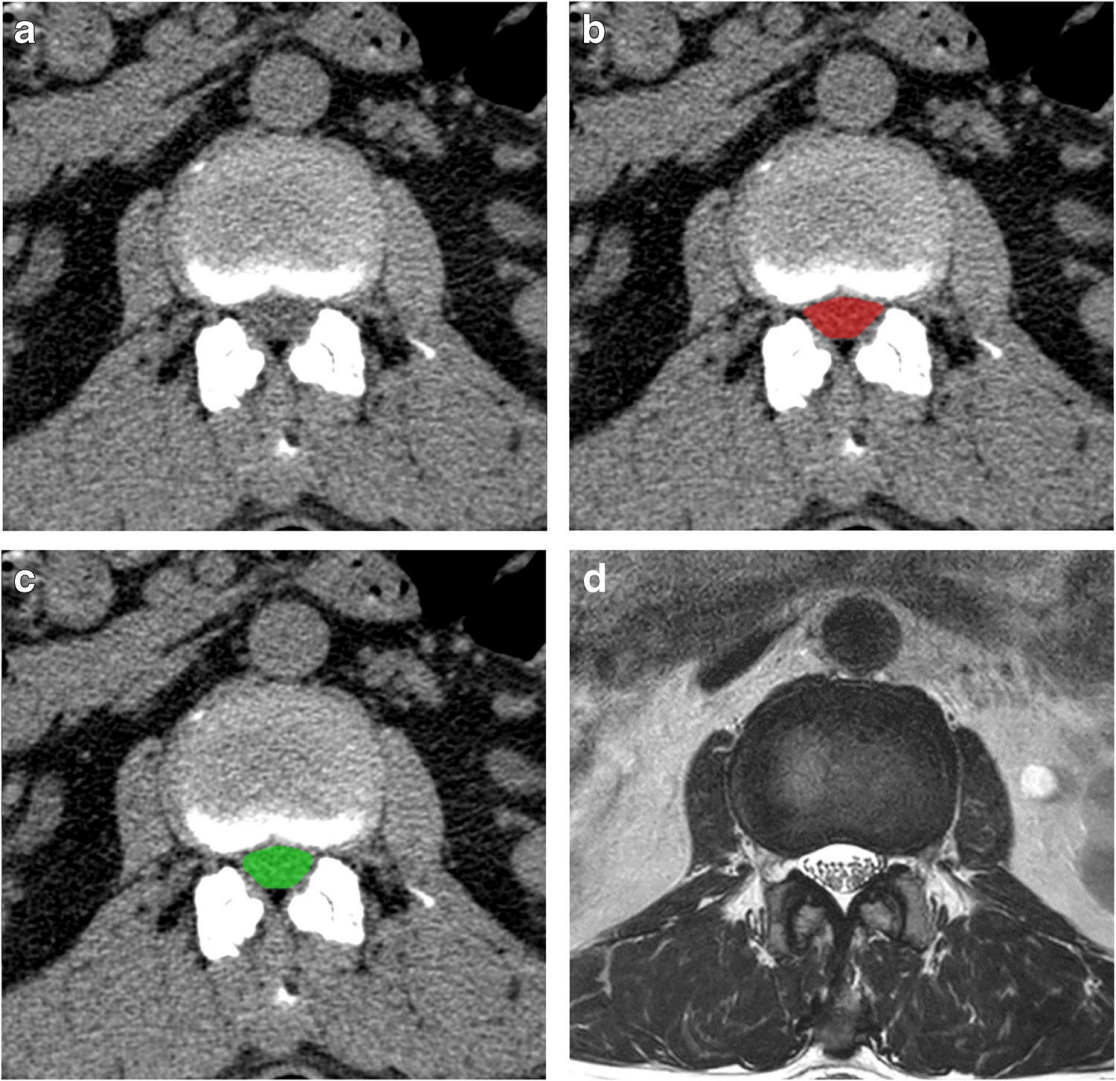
Representative cases of dural sac segmentation on a normal case of lumbar CT without contrast. The images of axial CT imaging (a) selected from the dataset are shown alongside their resulting segmentation of the dural sac by a manual rater (b, red) and the proposed algorithm (c, green). The dural sac area is 127.6 mm^2^ (b) and 124.2 mm^2^ (c), respectively. Figure 5d is an MR axial image of this case, which was taken within 6 months of the CT scan
